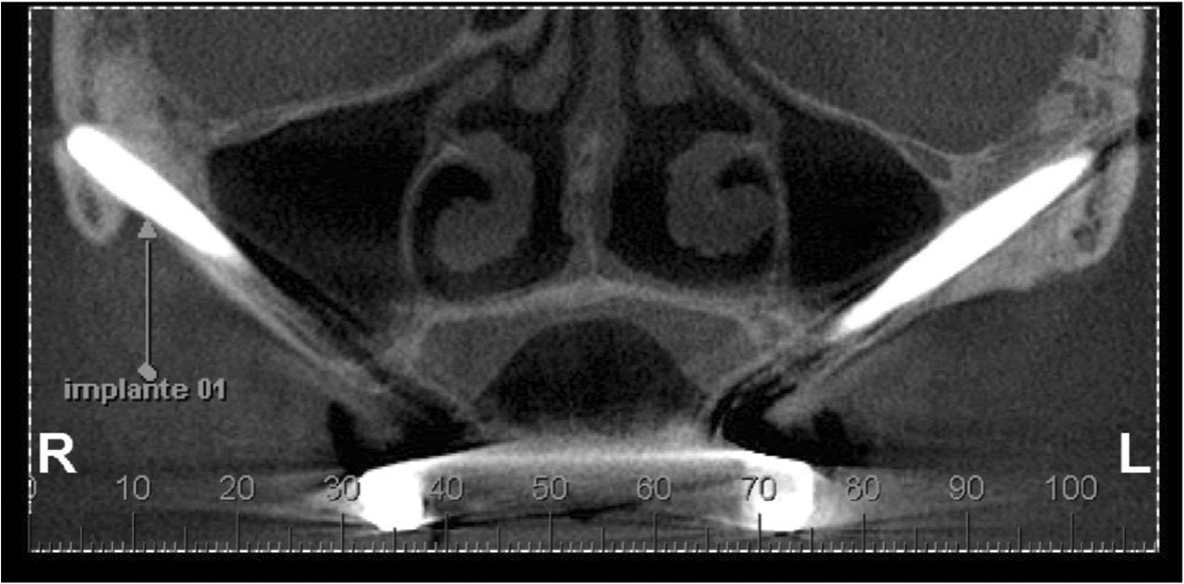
Coronal slice from the CBCT showing implant apical third inside the zygomatic bone
